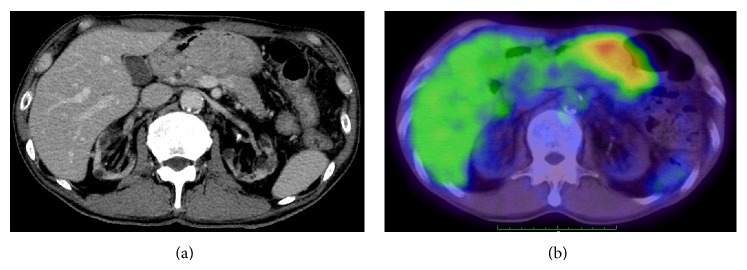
(a) A computed tomography scan revealing a slightly enhanced lobular tumor (34 mm × 54 mm) protruding into the duodenum bulb. (b) Positron emission tomography showing an accumulation of ^18^F-fluorodeoxyglucose (60 mm × 34 mm, maximum standardized uptake value = 5.6).
